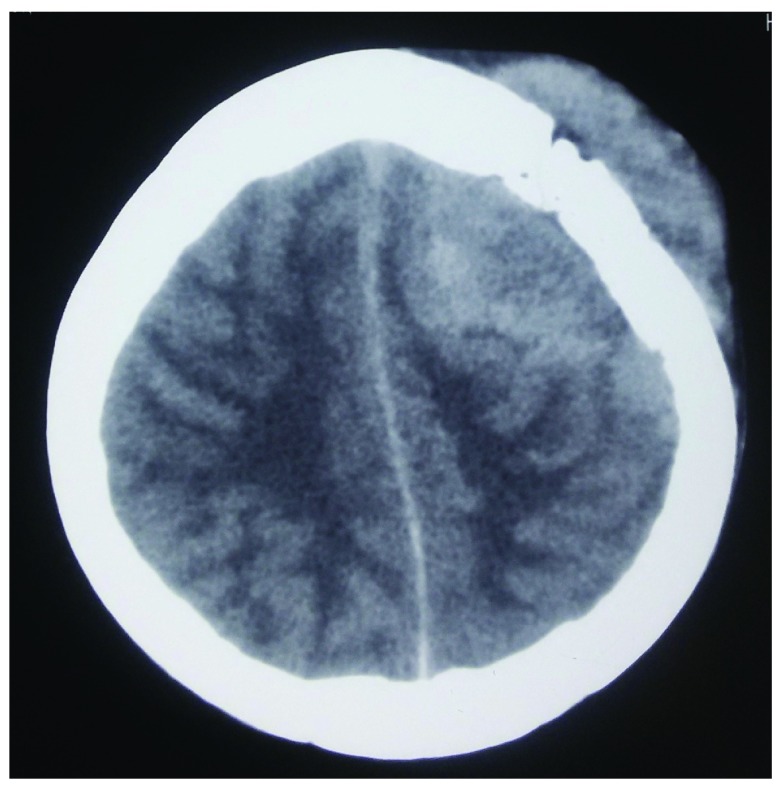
CT image showing hyperintense lesion surrounding the skull bone.
